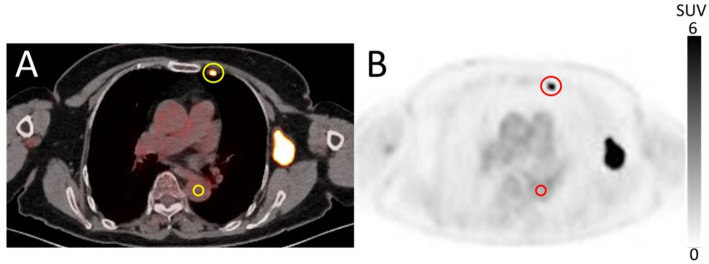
Representative VOI placements on the clinical A PET/CT and B PET images. A VOI of sufficiently large size was placed on the metastatic IMLN tumor, and a VOI of 1.5 cm in diameter was placed in the descending aorta (as the background)
